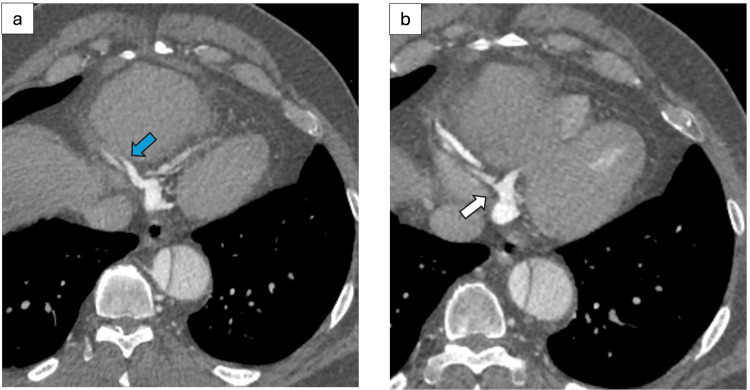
Axial Computed Tomography Images Showing Dilated Right Cardiac Vein and Coronary Sinus Ostial Narrowing (a) Axial contrast-enhanced computed tomography shows dilated right cardiac vein (blue arrow), and (b) axial contrast-enhanced computed tomography at the level of the inferior heart demonstrates coronary artery ostial narrowing (white arrow).
